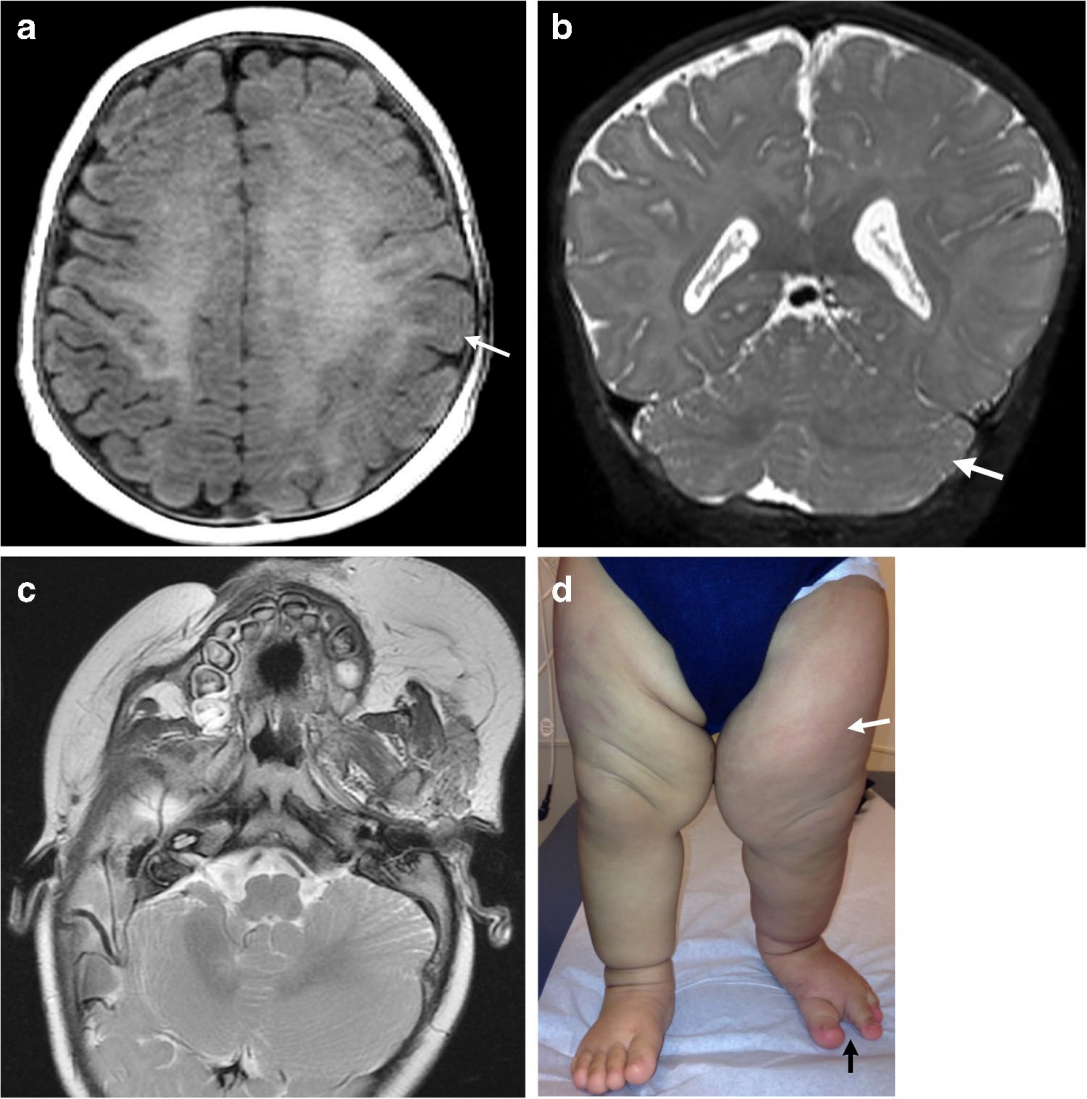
CLOVES syndrome in a 5-month-old boy with genetically confirmed *PIK3CA* mutation with mild hemimegalencephaly and a normal head circumference. a An axial spoiled gradient recalled echo image shows mild hemimegalencephaly and subtle pachygyria in the left perirolandic region (*arrow*). b A coronal T2-weighted image shows associated enlargement of the left cerebellar hemisphere (*arrow*). c An axial T2-weighted image shows lipomatous overgrowth of the left facial soft tissues. d A clinical photograph shows subtle overgrowth of the left lower extremity with associated capillary malformations (*white arrow*) and a sandal gap deformity (*black arrow*)
